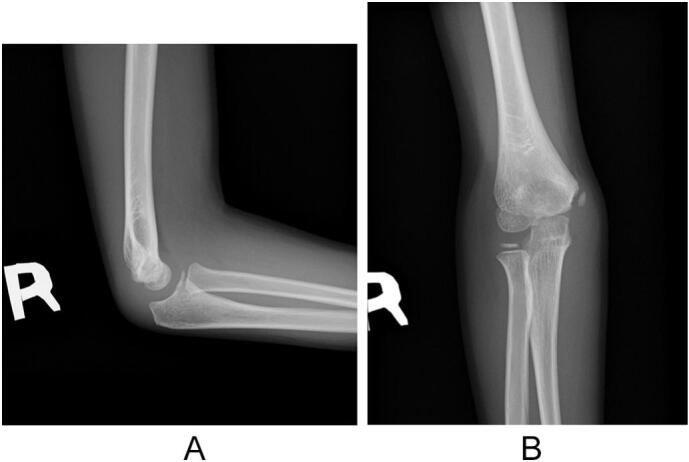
Plain X-ray images at 1 year after surgery. (a) Anteroposterior view. (b) Lateral view.
